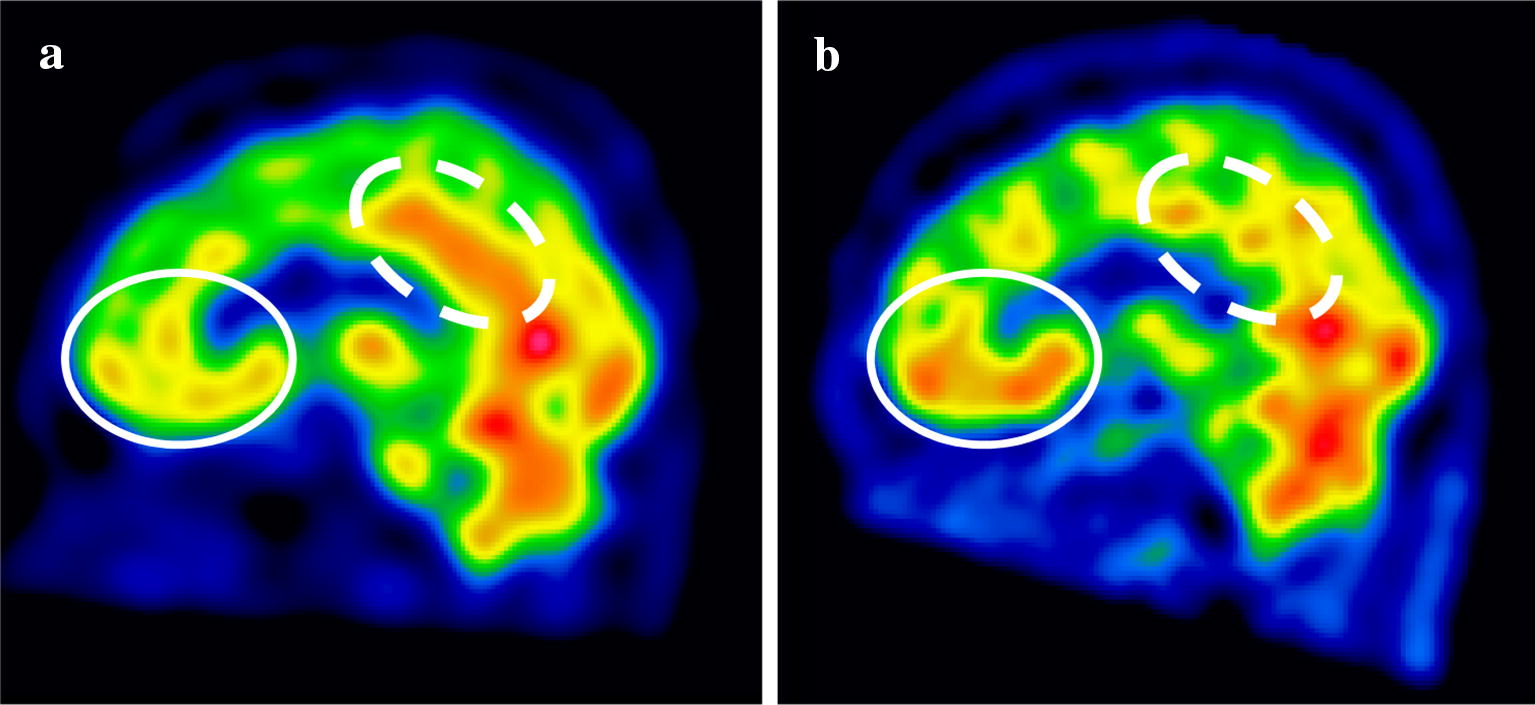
Cerebral blood flow imaged by SPECT during mania and after recovery. a During mania, the blood flow in the orbitofrontal cortex (OFC) was low (solid-line circle), while the blood flow in the posterior cingulate cortex (PCC) was high (dashed circle). b After recovery, blood flow was normalized in both the OFC and the PCC
